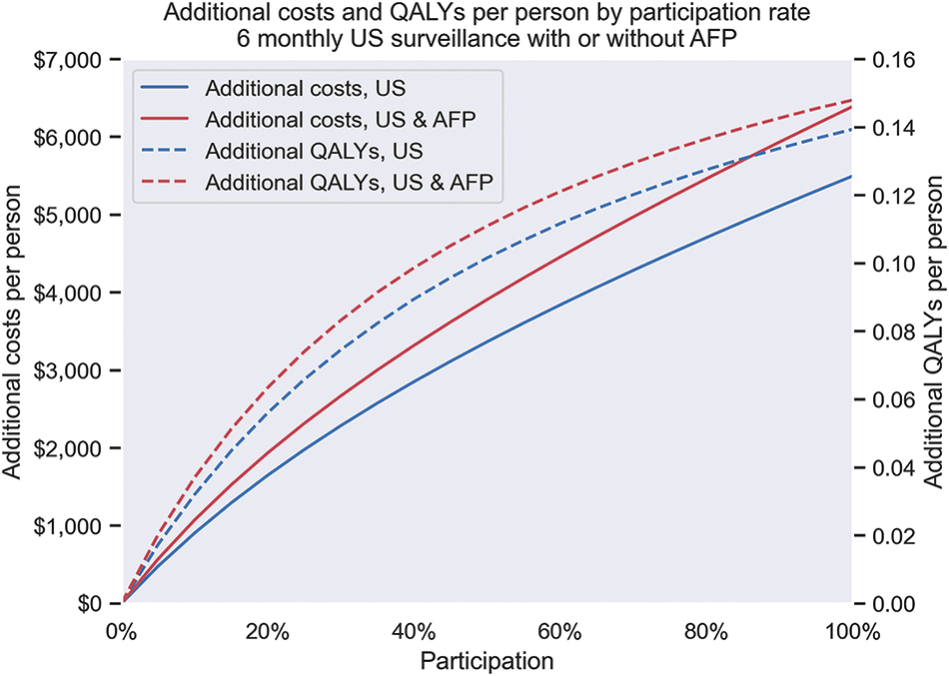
Health benefits and costs associated with 6-monthly surveillance, by level of adherence. Comparator: no surveillance. AFP, alpha-fetoprotein; QALYs, quality-adjusted life-years; US, ultrasound.
